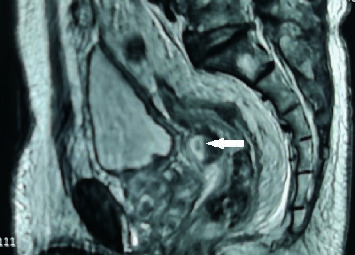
Abscess shrank with low signal in its center (white arrow) after MRI reexamination.
